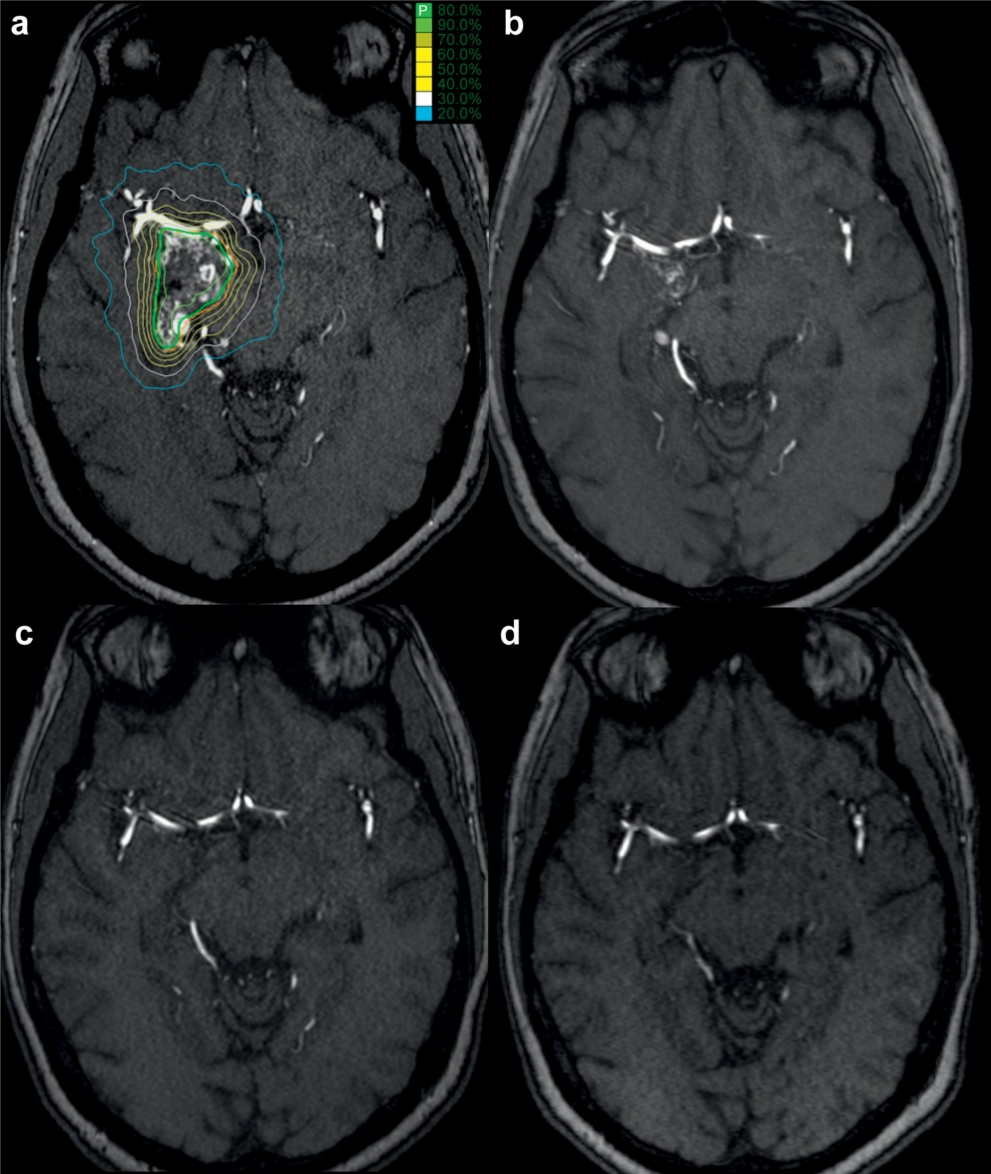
Exemplary obliteration process after CyberKnife radiosurgery. An example of the obliteration process for one patient with Spetzler-Martin grade IV arteriovenous malformation (AVM). The images show the CT scan with the CyberKnife treatment plan (red: target, green: prescribed isodose) a MR angiography (a), the MR angiography scans 8 months after treatment showing partial obliteration (b), and MR angiography scans of the same area two years (c) and five years (d) after radiosurgery, demonstrating complete obliteration of the AVM.
